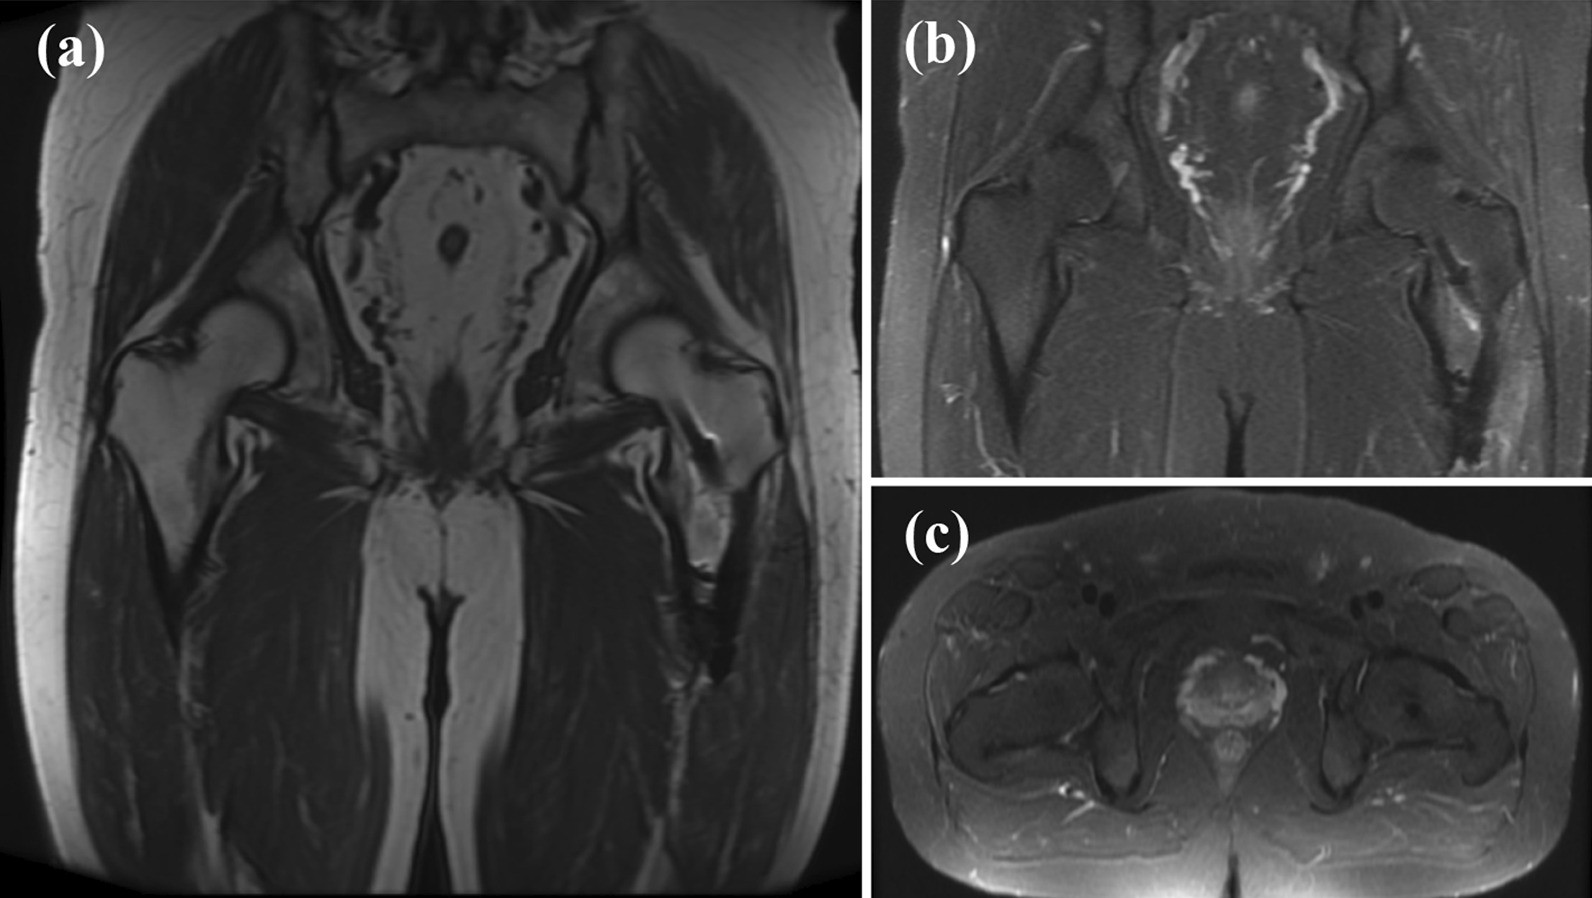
MRI performed 7 months after surgery. Coronal view (a) of hip joint T1-weighted MRI. Coronal view (b) and axial view (c) of hip joint short tau inversion recovery MRI. The fracture line in the proximal femur had disappeared
